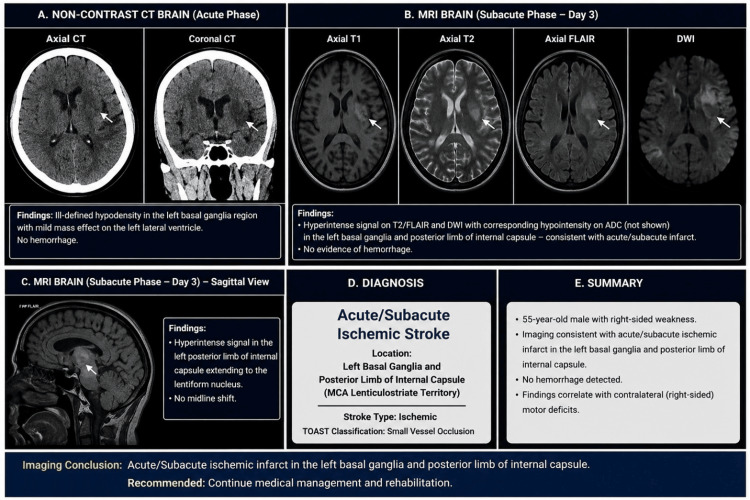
CT/MRI representation demonstrating ischemic stroke involving the left basal ganglia and posterior limb of the internal capsule, consistent with the patient’s right-sided motor deficits. FLAIR, fluid-attenuated inversion recovery; DWI, diffusion-weighted imaging; ADC, apparent diffusion coefficient; MCA, middle cerebral artery.
